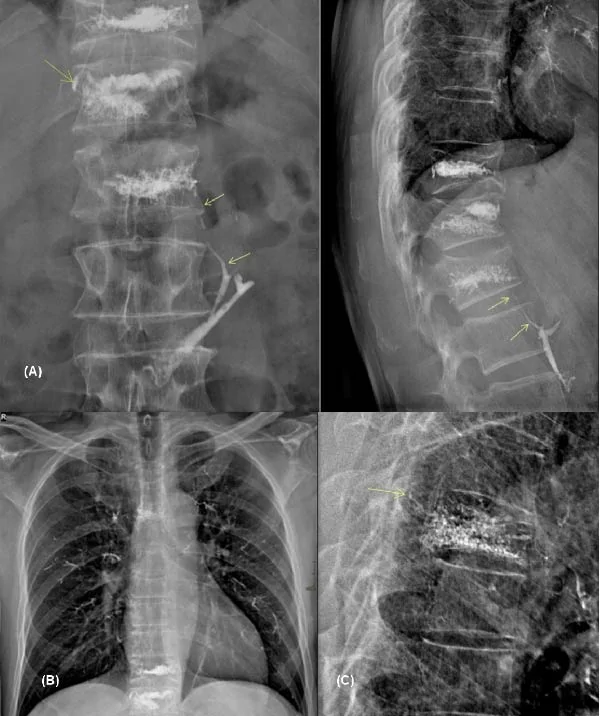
Radiographic images of the thoracolumbar spine following vertebral augmentation. (A) Anteroposterior and sagittal lumbar radiographs demonstrating vertebroplasty/kyphoplasty cement opacification at T11, T12, and L1 (yellow arrows) with no posterior wall retropulsion. (B) Frontal chest radiograph showing cemented T5 vertebral level (yellow arrow). (C) Magnified lateral thoracic view confirming intact posterior vertebral wall and stable cement distribution at T5.
